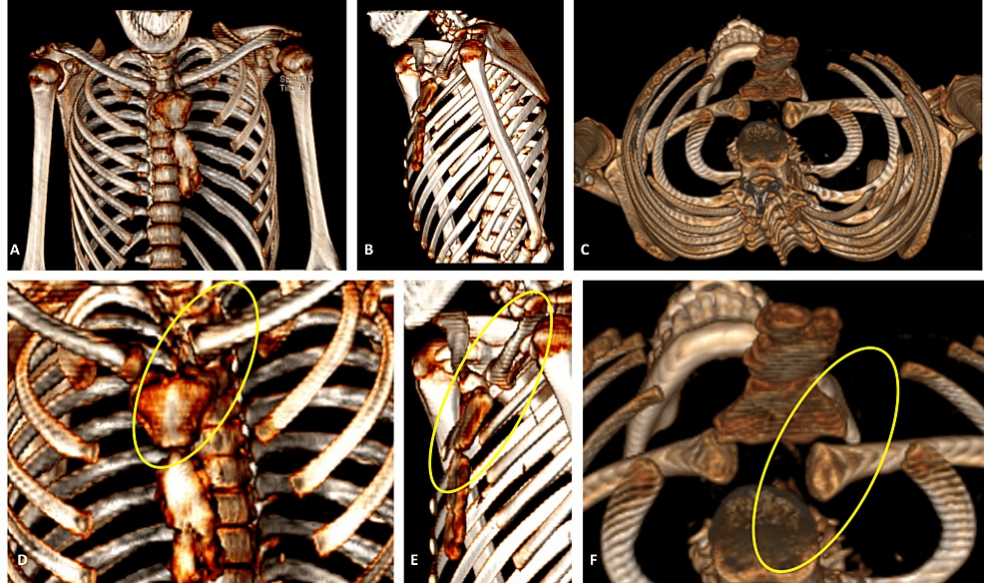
Three-dimensional reconstructed computed tomography of the patient after the trauma before the closed reduction Anterior (A), lateral (B), inferior (C), magnified anterior (D), magnified lateral (E), and magnified inferior (F) views. The left sternoclavicular joint is marked with a yellow ellipse.
